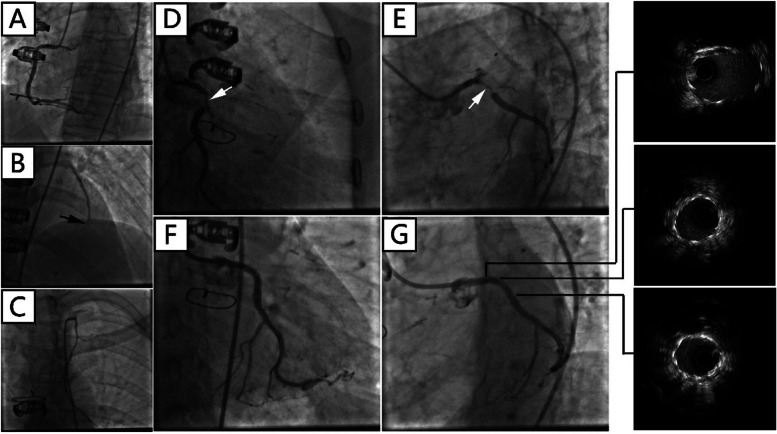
Coronary angiography on May 25, 2021. Blood flow in right coronary artery (A) and left internal mammary artery to first diagonal branch bypass graft (C) were unobstructed; (B) Great saphenous vein graft to left anterior descending coronary artery was totally occluded (black arrow); (D) and (E) Index angiogram showed severe in-stent stenosis in proximal left circumflex coronary artery (white arrow); (F) and (G) Angiogram and intravascular ultrasound post drug-coated balloon showed optimal stent expansion with no malapposition
